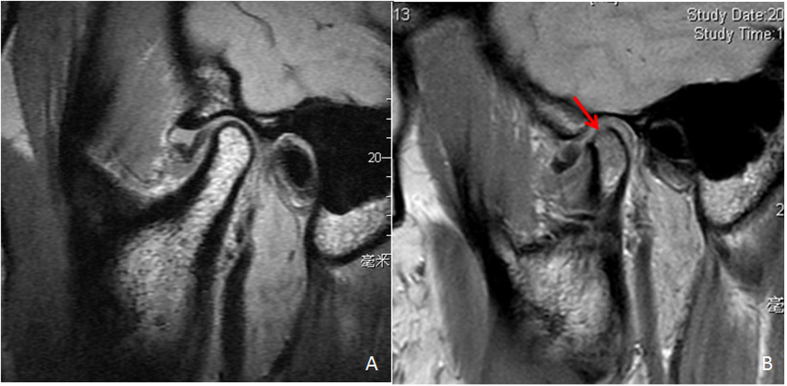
MRI showed condylar bone degeneration after ATDD. (A) disc displaced anteriorly 3 weeks after injury, the condylar bone was intact. (B) the condylar surface bone resorbed 5 months after injury (arrow).
